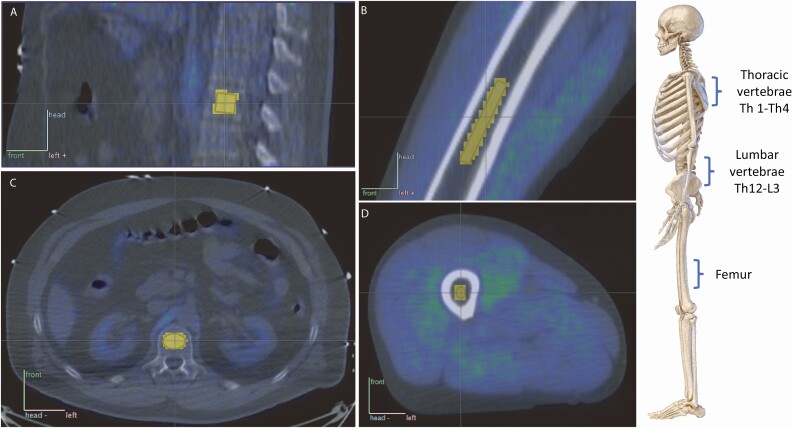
An example of the shape and positioning of the region of interest (ROI) from which time activity curves were extracted. Sagittal PET/CT image of lumbar vertebral (A) and femoral (B) regions. Transaxial PET/CT image of lumbar vertebral (C) and femoral (D) regions. CT scans were used as anatomical reference.
